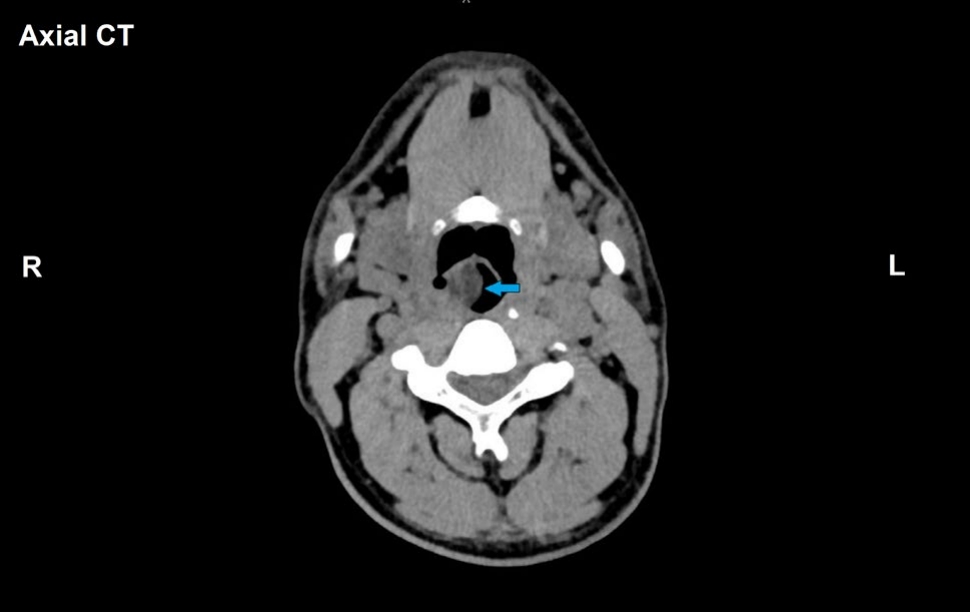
Radiological features of the hypopharyngeal well-differentiated liposarcoma with prominent myxoid stroma. Axial section CT-scan of the neck demonstrates well-defined lesion with clear fluid density contents along the posterior wall of the hypopharynx, encroaching upon the hypopharyngeal airway (blue arrow) (Color figure online)
